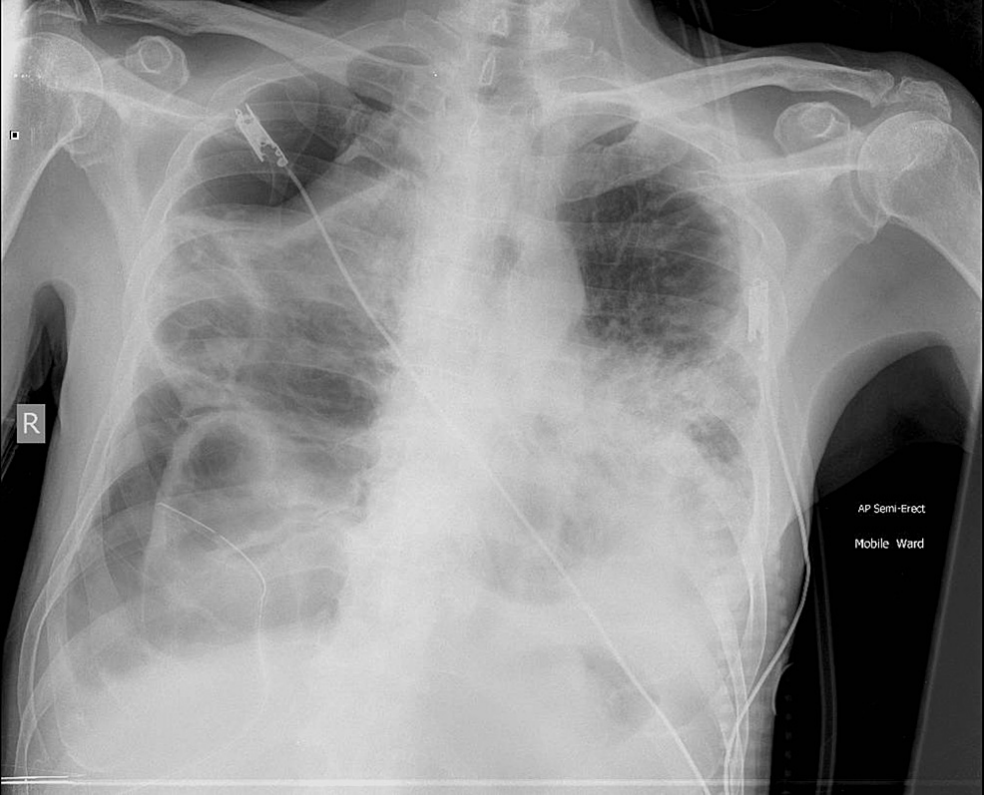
One hour post chest drain insertion chest X-ray on day 1 of current admission
